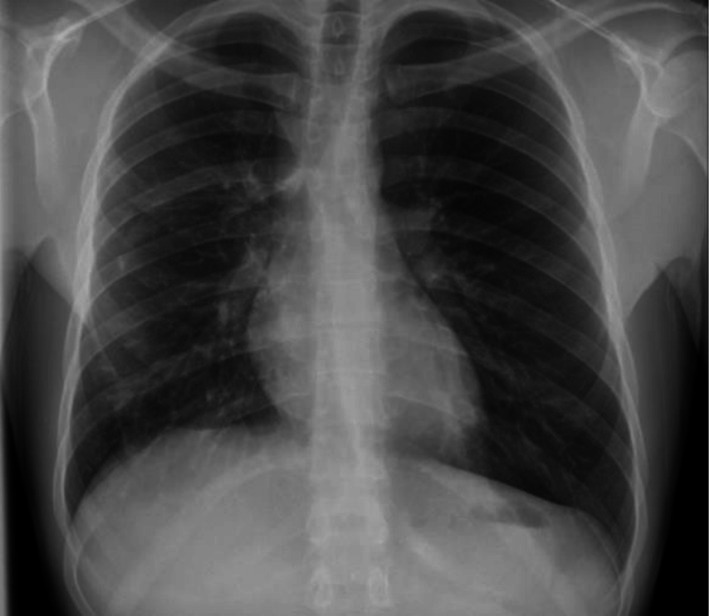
Follow‐up chest radiograph showing near‐complete resolution of bilateral infiltrates after cessation of mesalazine and initiation of systemic corticosteroid therapy.
